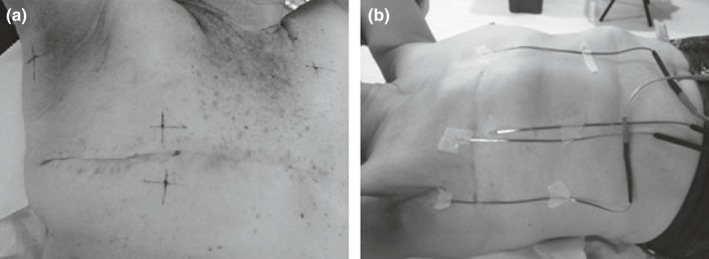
Patient skin measurement points tattooed during the computed tomography scan (a). Skin MOSFETS positioning on the patient prior treatment (b).
